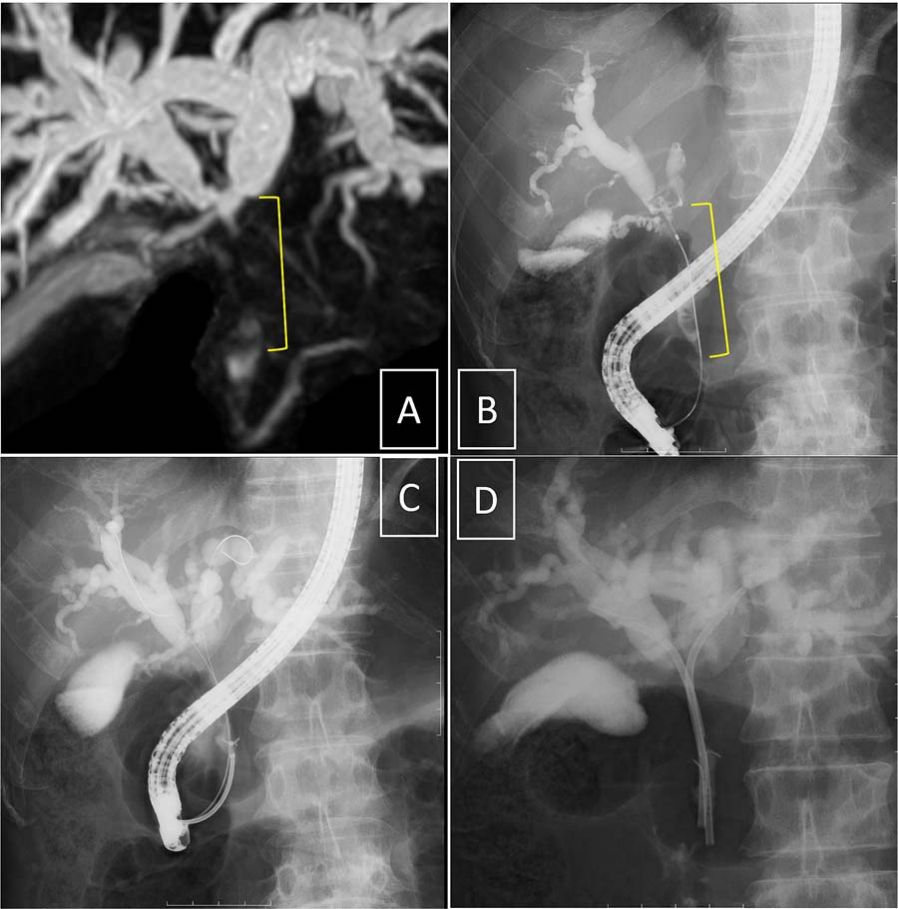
Cholangiography. Magnetic resonance imaging (A) and endoscopic retrograde cholangiopancreatography (B, D) reveal full circumferential stenosis in the mid-to-upper bile ducts without left and right bile disconnections. (C) The biopsy is conducted in the distal bile duct.
